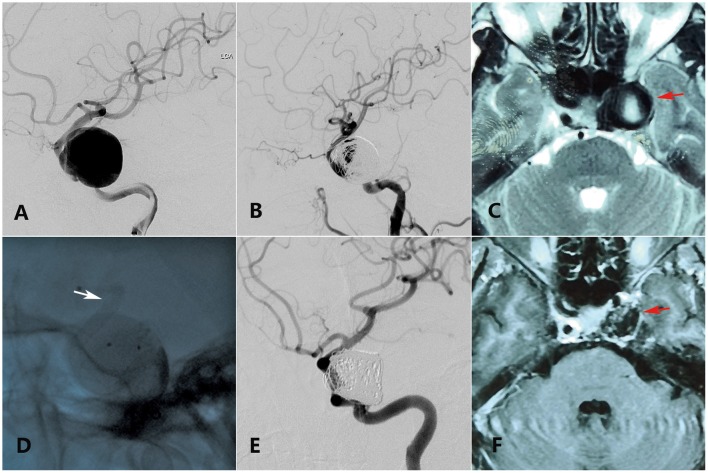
A 55-year-old woman with a giant ophthalmic segment ICA aneurysm presented with visual loss and incomplete ONP of the left side showing visual improvement merely in the last clinical follow-up. (A) Preoperative DSA shows a giant ophthalmic segment ICA aneurysm. (B) Immediate postoperative DSA shows loose embolization of aneurysm. (C) Preoperative axial-plane MRI shows an ophthalmic segment ICA aneurysm (red arrow). (D) Intraoperative DSA shows placement of a PED (white arrow) covering the aneurysmal neck. (E) Thirteen-month DSA shows variation of the coils in the sac. (F) Twenty-five-month axial-plane MRI shows shrinkage of the aneurysm (red arrow).
